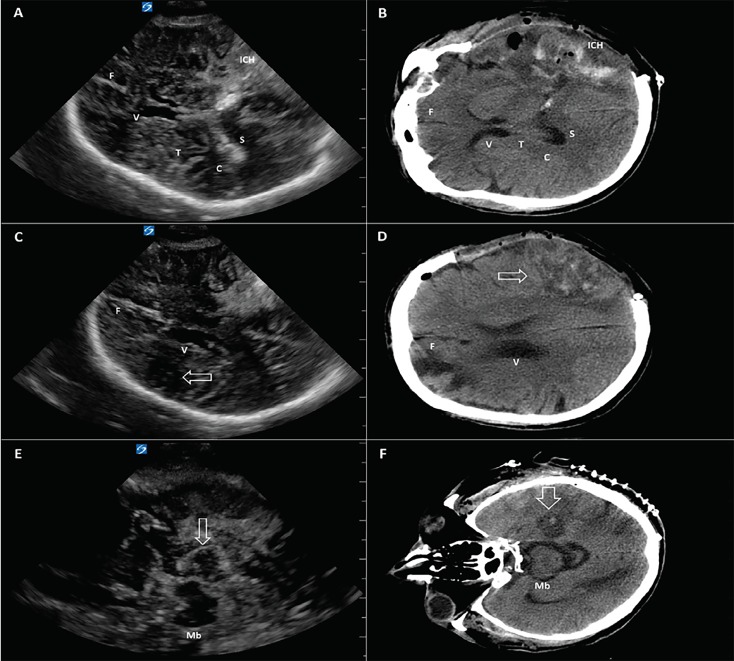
Cranial ultrasound through the left transtemporal window (left column, panels A, C, E), with corresponding cross-sectional anatomy on a non-contrast computed tomography of brain (right column, panels B, D, F) in a 74-year-old male with a left hemicraniectomy. Intracranial hemorrhage (ICH) and hypodense ischemic areas are indicated by white arrows. *F*, falx cerebri; *V*, lateral ventricles; *Mb*, midbrain; *C*, choroid plexus; *S*, splenium of corpus collosum; *T*, thalami.
